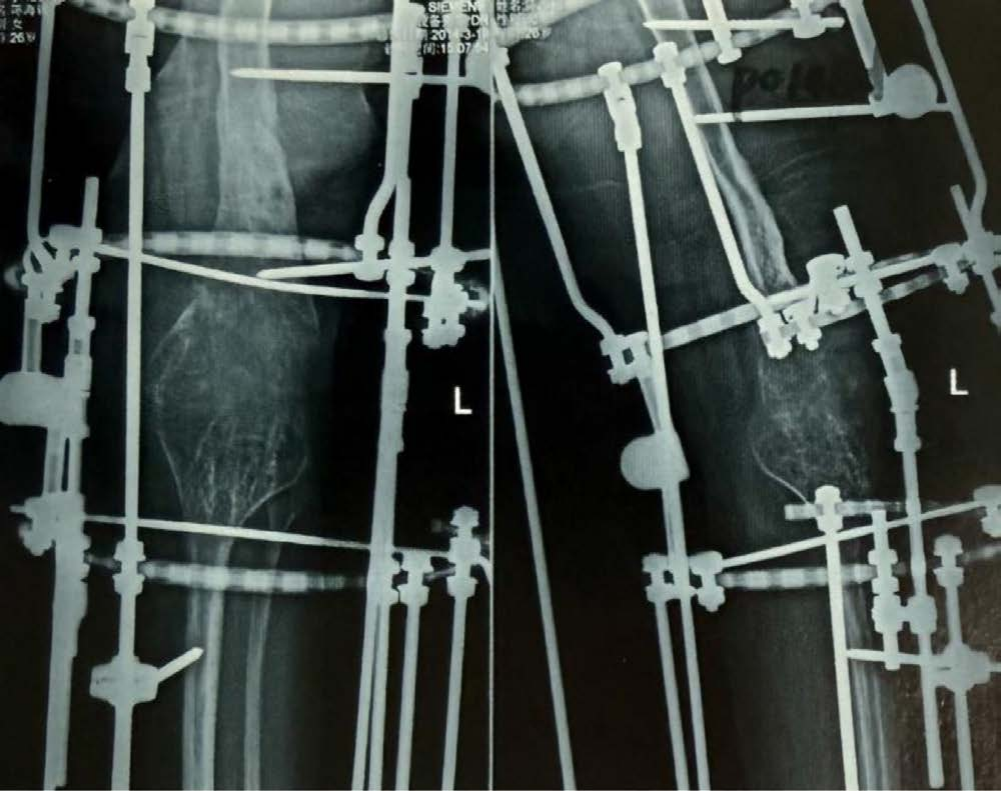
X-ray examination at 128 days postsurgery showing good bone healing at the knee osteotomy site.
